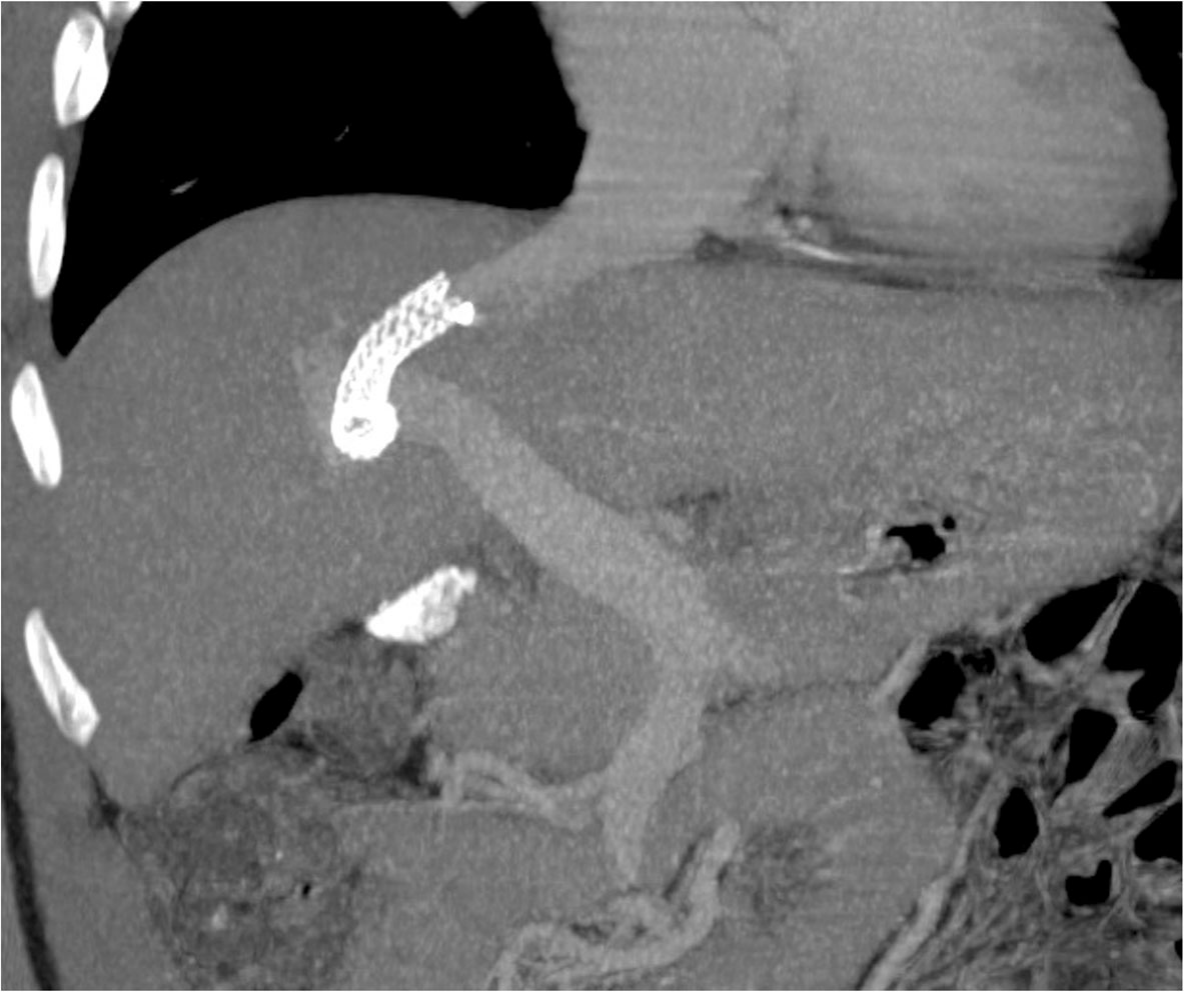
Transjugular intrahepatic portosystemic shunt. MIP image from portal venous phase CT shows a metallic stent connecting the PV with the hepatic vein. Note enlargement of the hepatic vein due to increased flow directly drained from the PVS to its lumen, skipping the hepatic parenchyma
